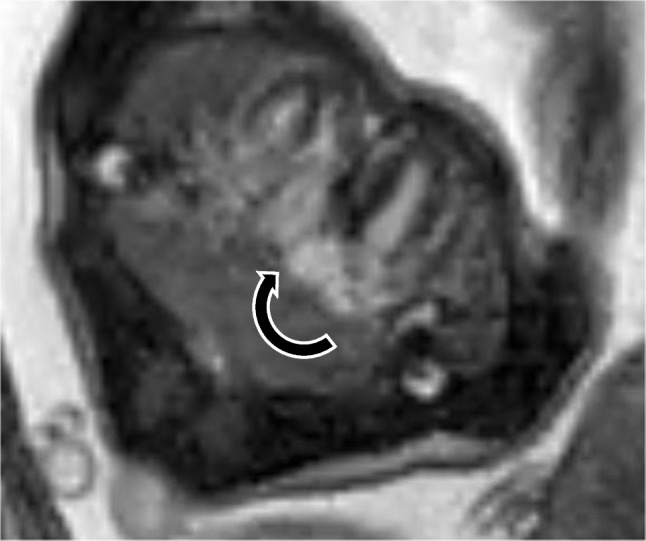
Fetal MRI (BTFE) of dicephalic parapagus at gestational age 25 weeks, 1 day, axial to the conjoined chest shows fused atria (*curved black arrow*). *BTFE*, balanced turbo-field echo
